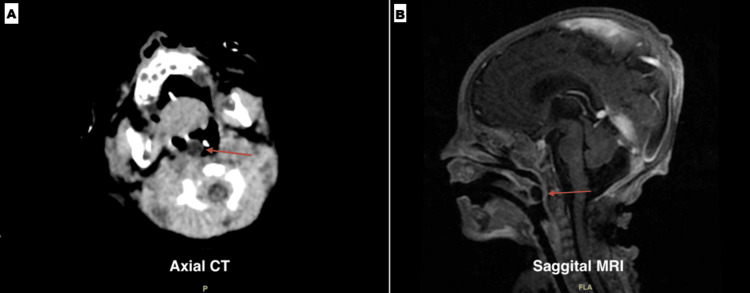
Axial CT (A) and sagittal MRI (B) images demonstrating a well-circumscribed, non-enhancing cystic lesion (arrows) within the uvula. The contents appear homogenous and fluid-filled, consistent with a benign cyst CT: computed tomography; MRI: magnetic resonance imaging
